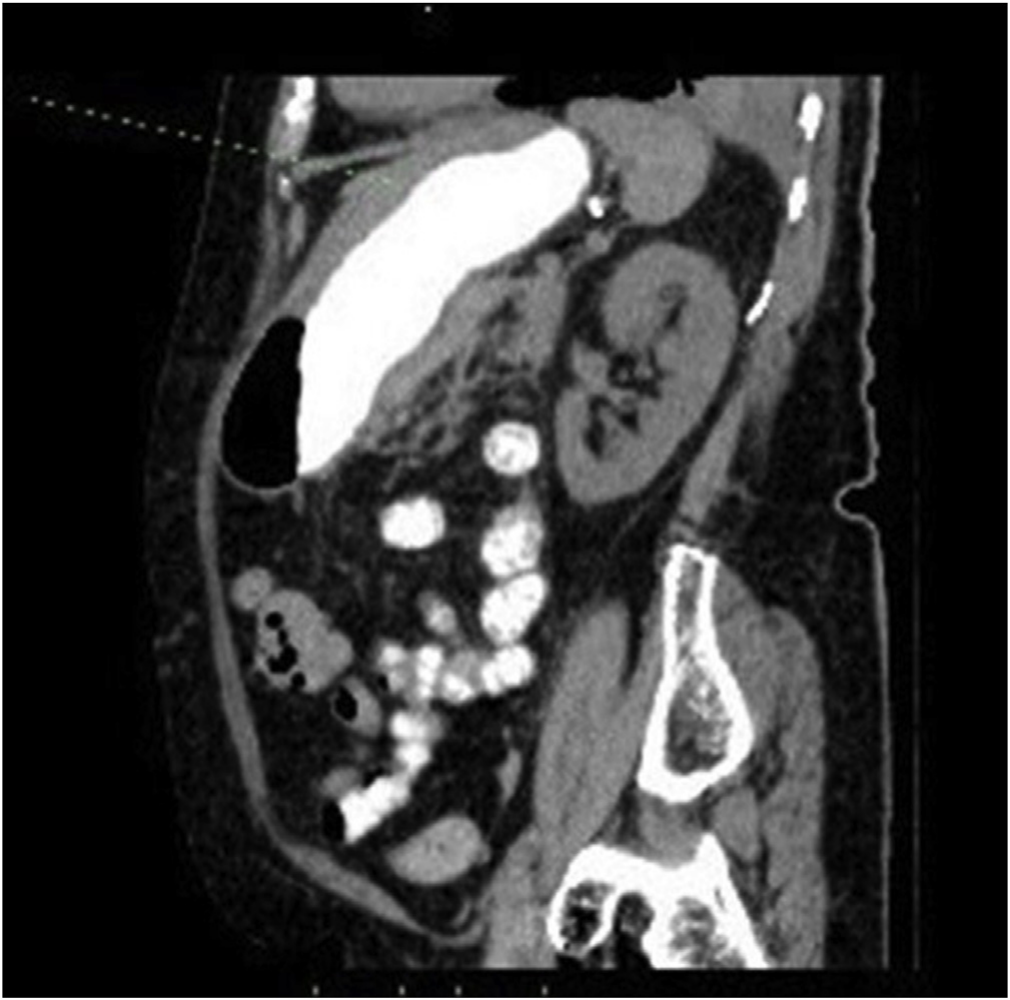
Parietal hematoma of the corpus and fundus on computed tomography.
